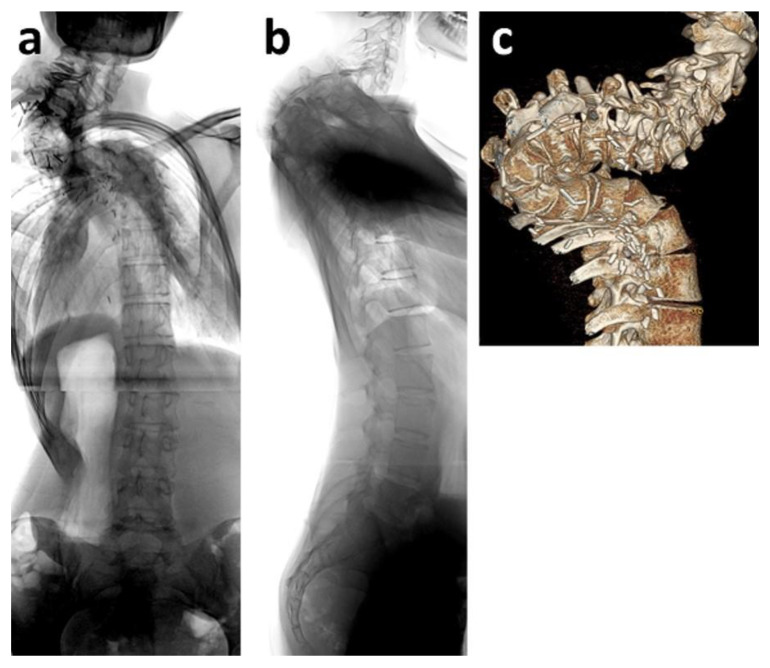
Fifteen-year old girl with juxta-spinal neurofibromatosis and severe three-dimensional upper thoracic spinal deformity (a–c). MRI prior to surgical treatment revealed severe neurofibromatosis with thoracic obstruction, which was incompletely removed.
